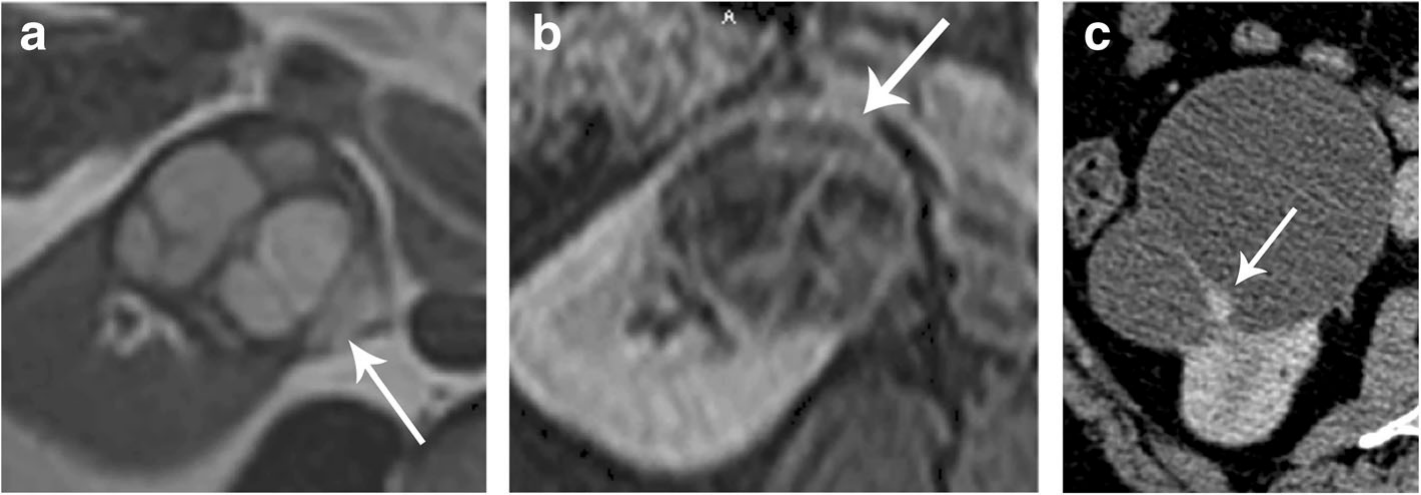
Bosniak IIIs (a, b) and IIIn (c) lesions. a Axial T2-weighted MR showing a multiseptated cystic renal lesion (arrow) and (b) axial T1 post-contrast MR of the same lesion showing septal enhancement (arrow) consistent with a Bosniak IIIs cyst. c Axial contrast-enhanced CT of a cystic renal mass with septae nodularity (arrow) considered to be a Bosniak IIIn cyst
